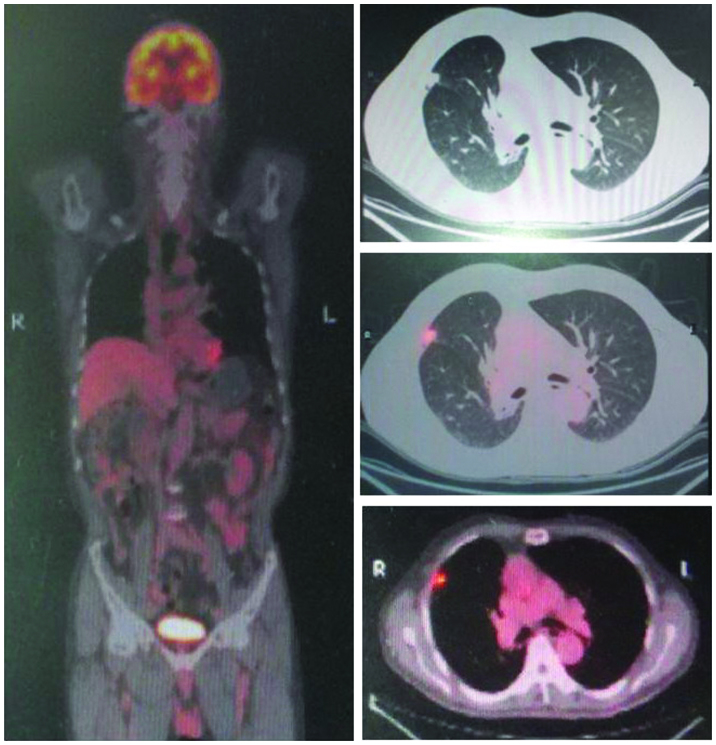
Positron emission tomography-computed tomography scans showing an abnormality with increased ^18^F-fluorodeoxyglucose uptake at the surgical site.
